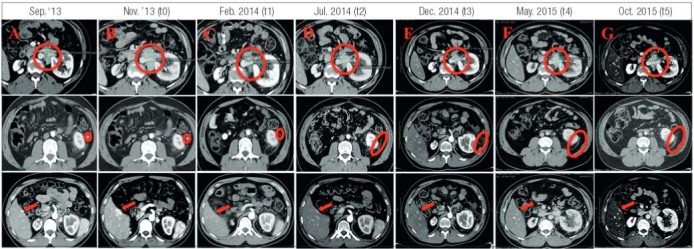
CT scan before sunitinib therapy: Sept. ’13 (A) and Nov.’13 (B), and during the follow up: t1 (C), t2 (D), t3 (E), t4 (F) and t5 (G). In the first line the main abdominal lesion, in the second line another abdominal lesion and in the third line liver metastases.
